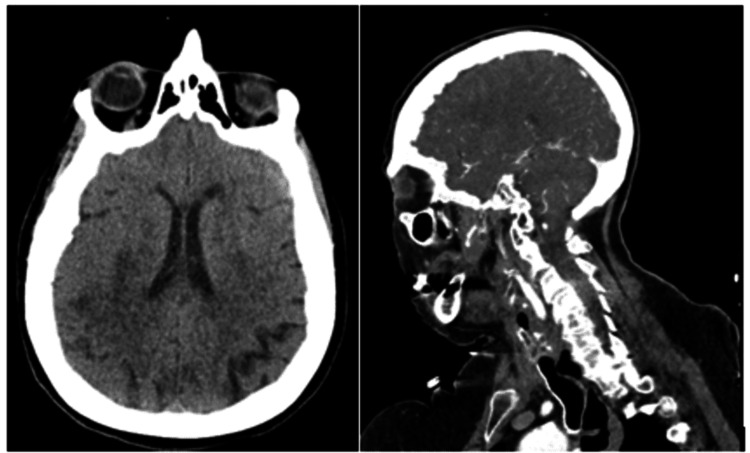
CT angiography of the head and neck The left panel shows the head, while the right panel displays the neck.
